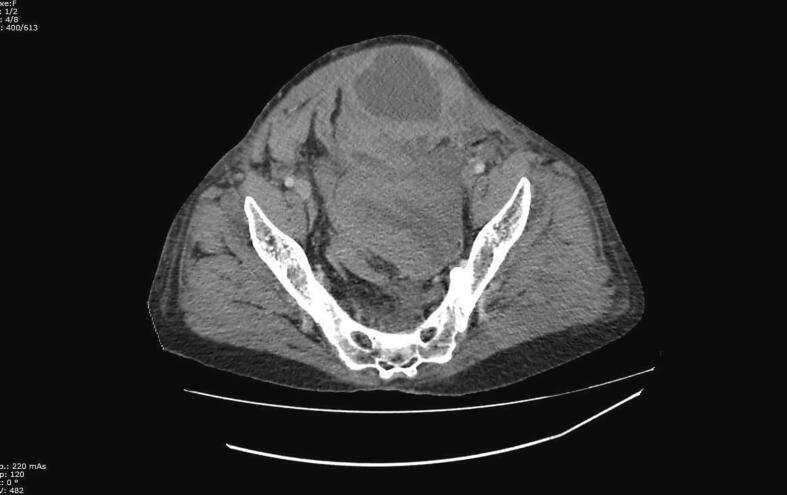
Axial section of a CT scan showing the large hematoma in the left rectus muscle.
